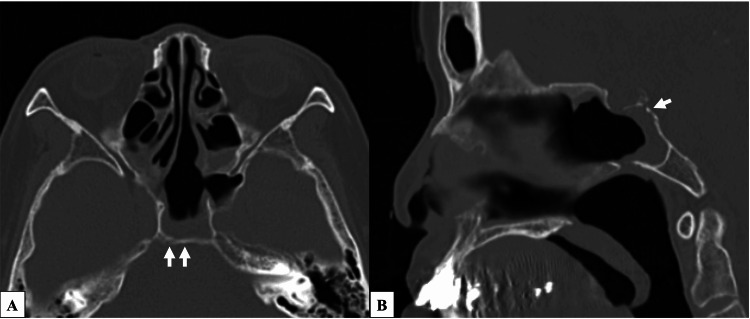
Computed tomography findings around the clivus at one month after surgery. The bony defects were well reconstructed. White arrows indicate the areas where bony defects were identified.
